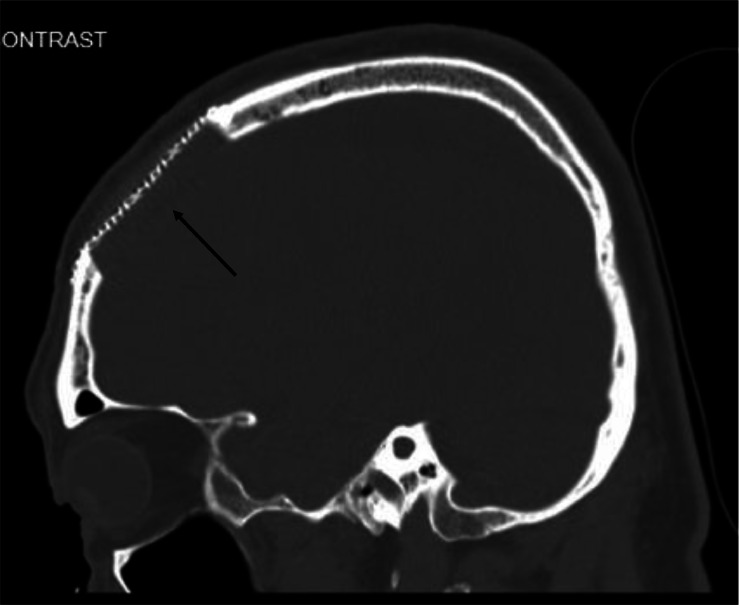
Post-op CT showing titanium plate over the cranial defect.
